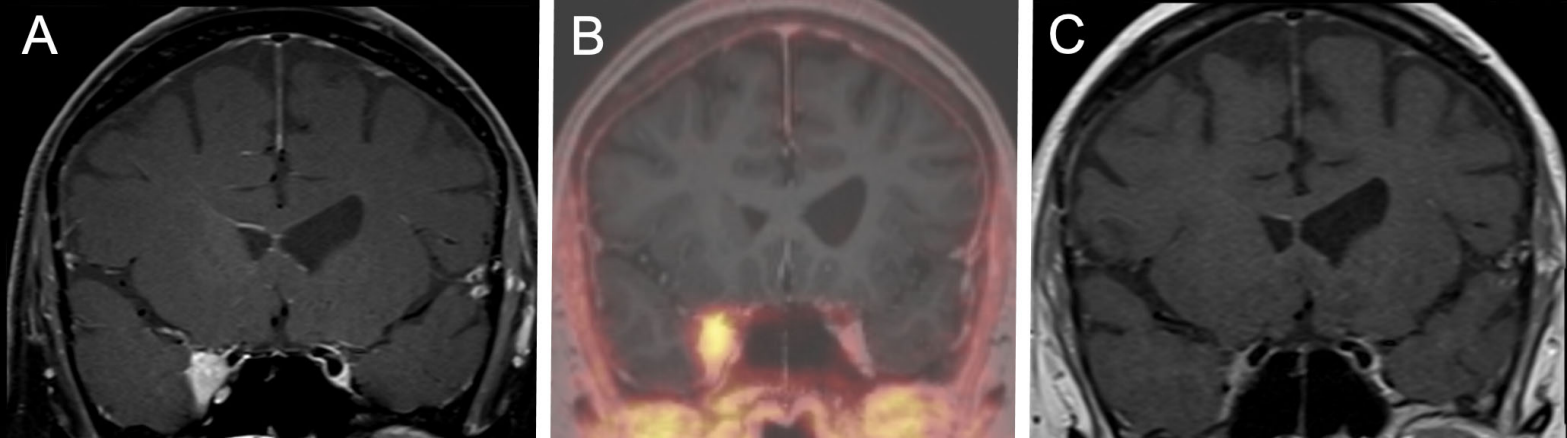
A representative case for the tumor response after hypofractionated SRS therapy. A 61-year-old female patient suffering from headaches and double visions. SRS was performed with a 5x5 Gray 70% isodose. (A) Coronal T1 weighted MRI scan after administration of Gadolinium prior to CyberKnife therapy showing a lesion infiltrating the cavernous sinus. (B) DOTATOC-PET/MRI Scan conforming a meningioma and enabling better target delineation. (C) Coronal T1 weighted MRI scan after administration of Gadolinium at the 18 months follow-up showing a complete remission of the lesion according to the RANO criteria. The patient reported a full remission of the headache and the double-visions at the follow-up.
